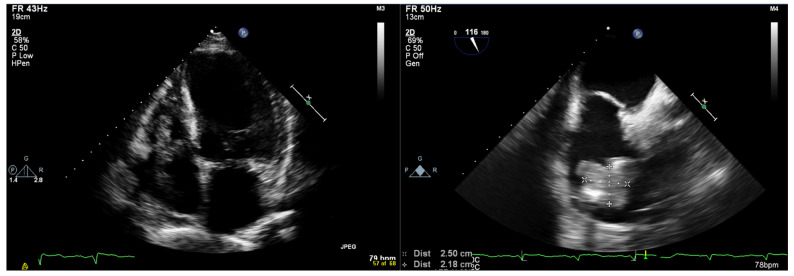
Ventricular pacing wire was inside the right ventricle with a large echogenic mass (2.2 × 2.5 cm) adherent to it. (Left) Transthoracic echocardiography study. (Right) Transesophageal echocardiography study.
